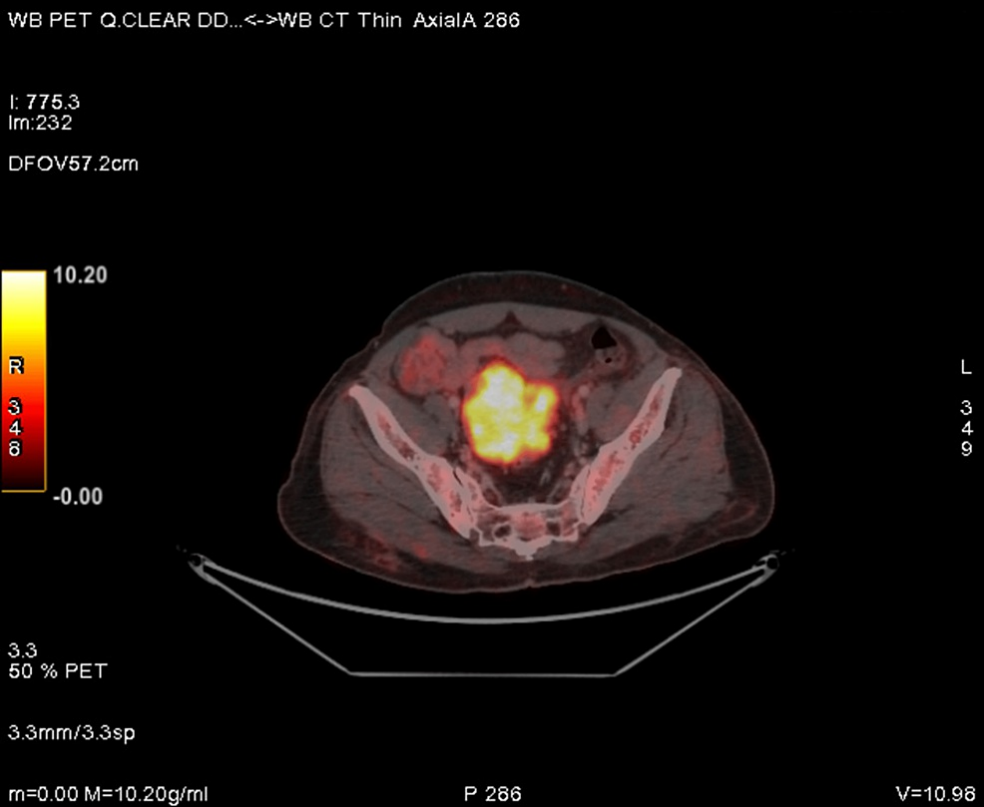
PET-CT showing FDG avid heterogeneously enhancing soft tissue density mass lesion in rectovesical pouch measuring 7.4 × 5.8 × 6.2 cm. FDG: 18-fluorodeoxyglucose; PET/CT: positron emission tomography/computed tomography
